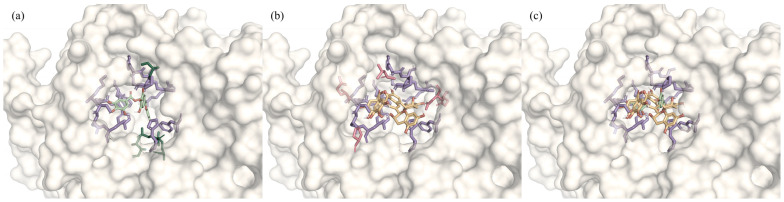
Structural basis underlying antagonistic interactions between EGCG and TFDG in α-glucosidase inhibition. (a) Predicted binding mode of EGCG (green) within pocket C1 of α-glucosidase, highlighting residues shared with TFDG (purple) and additional peripheral residues unique to EGCG (dark green); (b) predicted binding mode of TFDG (yellow), showing shared residues (purple) and additional peripheral residues unique to TFDG (rose); (c) overlay of EGCG and TFDG within pocket C1 of α-glucosidase, highlighting the conserved set of shared residues (purple).
